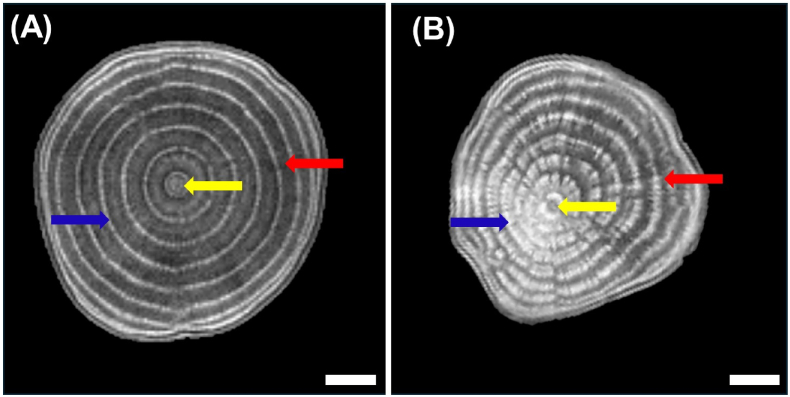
Comparison of MRI signal intensity and cross-sectional structures of (A) control taproot C1 and (B) diseased taproot D1 at 63 days after inoculation. Yellow arrows indicate central core, red arrows indicate storage parenchyma and blue arrows indicate cambium ring structure. Diseased taproot shows brighter and smeared ring structure with less phloem parenchyma area as compared to control taproot. Bars, 0.5 ​cm.
